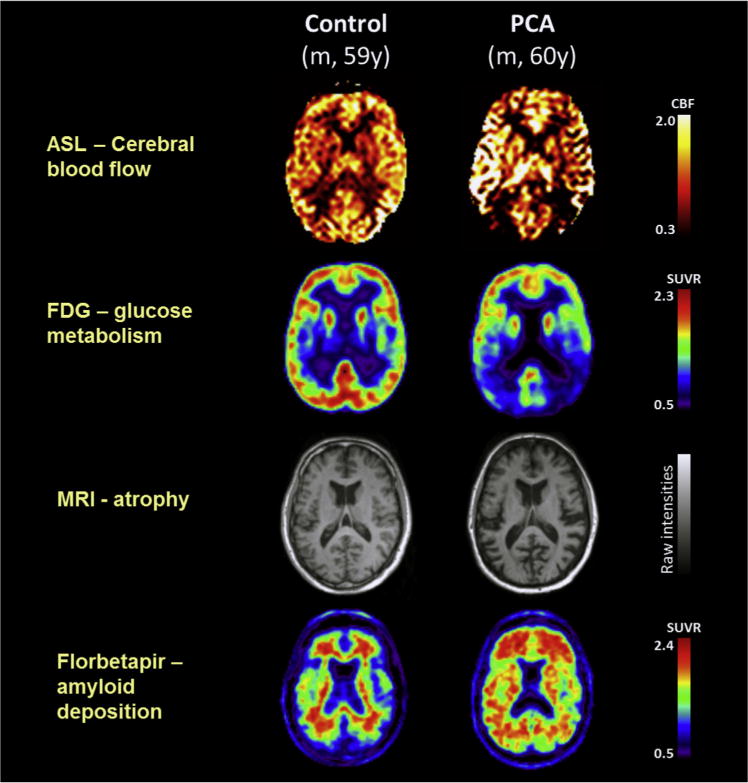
Single-participant axial images for one control participant and one patient with PCA showing cerebral blood flow (ASL), glucose metabolism (FDG-PET), atrophy (structural magnetic resonance imaging), and amyloid deposition (florbetapir-PET). For clinical purposes, ^18^F-florbetapir images should be read on a gray scale. Abbreviations: ASL, arterial spin labeling; CBF, cerebral blood flow; FDG-PET, ^18^F-labeled fluorodeoxyglucose positron emission tomography; PCA, posterior cortical atrophy; SUVR, standard uptake value ratio. For tau deposition data see Ossenkoppele et al [2]. Adapted from Lehmann et al., 2016, Figure 1.
